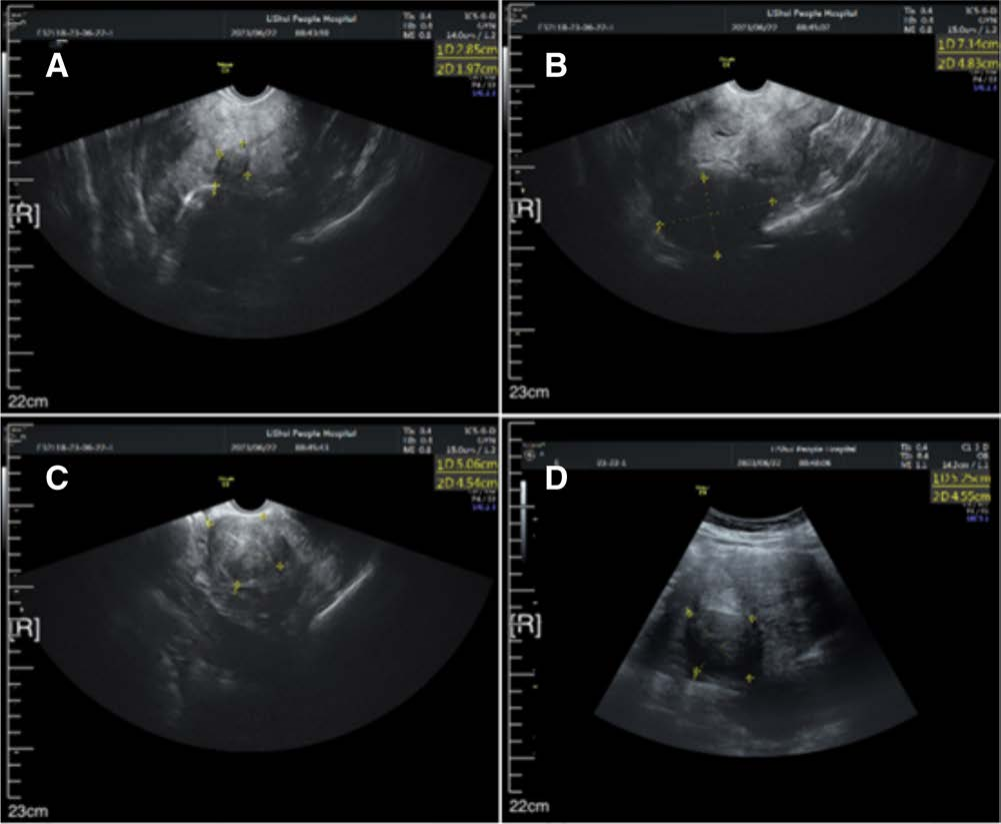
Transvaginal ultrasonography images. (A) Hypoechoic mass in the anterior wall of the uterus, about 29 × 20 mm in size; (B) Myomatoid mass fused with the posterior wall of uterus, 71 × 84 mm in size. (C, D) Another myomatoid mass located in the posterior wall, 51 × 46 mm in size.
